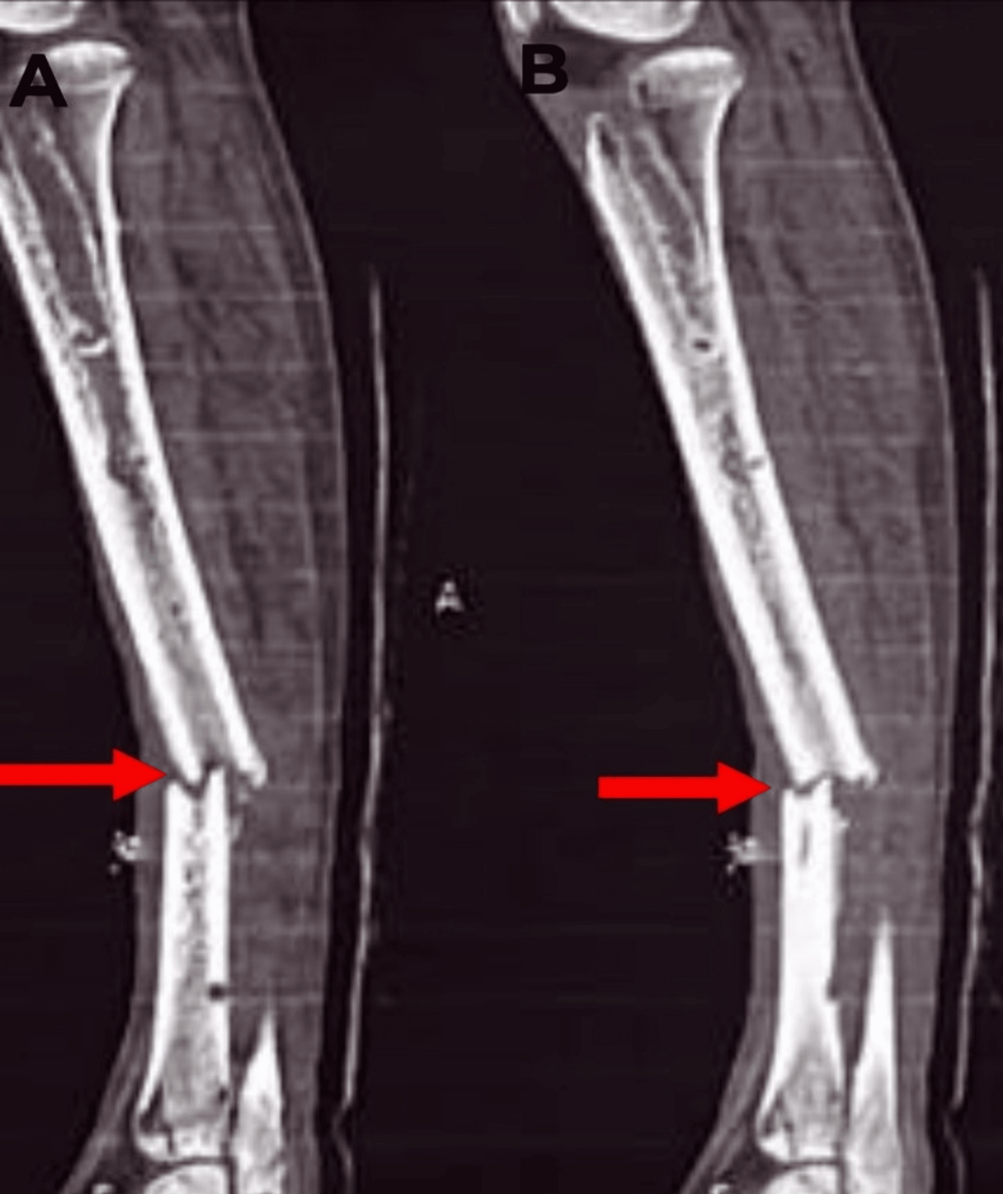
A-B) The sagittal section of the left leg CT scan. Red arrows show a complete, non-united displaced fracture in the middle one-third of the tibia shaft. CT: computed tomography
